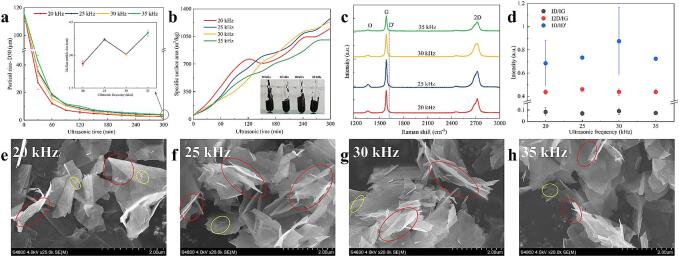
Change of FLG with ultrasonic time: a, particle size; b, specific surface area (the lower right corner is the FLG dispersion). FLG of ultrasonic 300 min: c, Raman spectrum; d, the ratio of Raman characteristic peaks. e-h, SEM images of FLG prepared at different frequencies. Red marks indicate rippled, crumpled, and curled areas; yellow marks highlight regions with crease lines and wrinkles. (For interpretation of the references to colour in this figure legend, the reader is referred to the web version of this article.)
